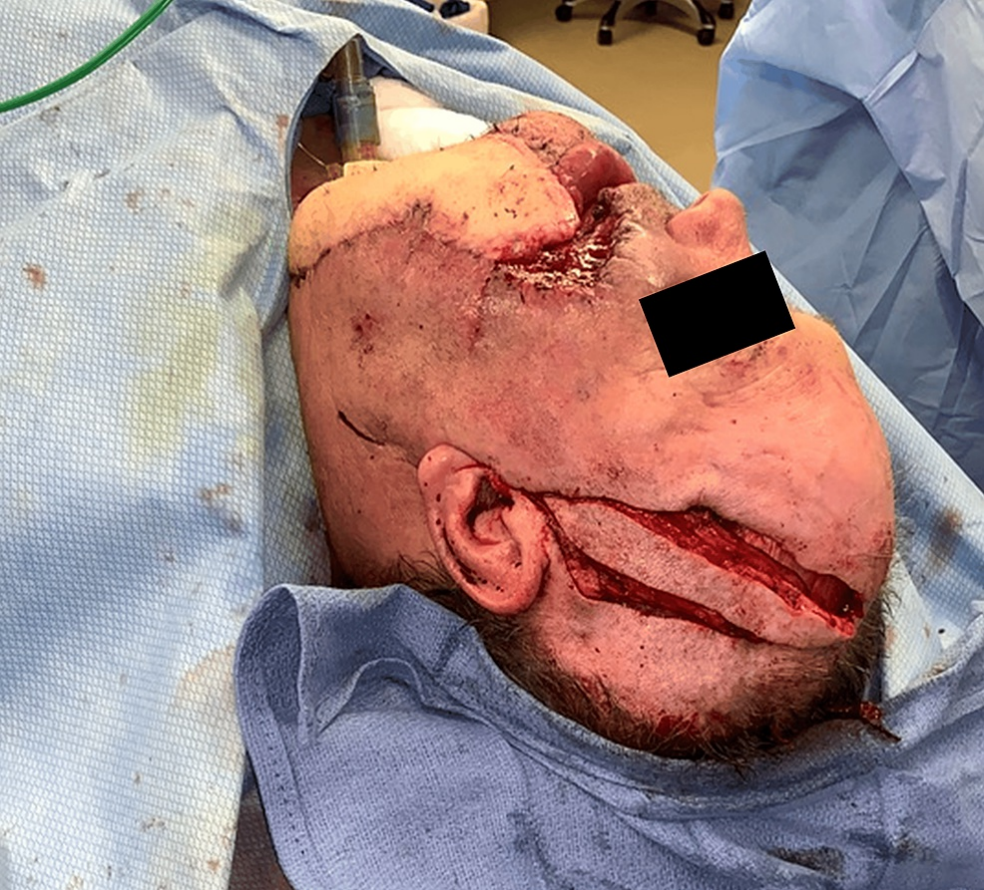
Harvest of the superficial temporal islandized artery flap (STAIF) pedicle following Doppler probe usage for mapping.
